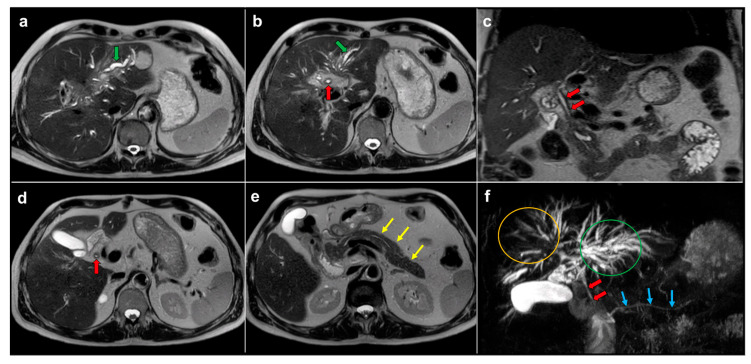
Primary sclerosing cholangitis in a male patient with ulcerative colitis and recurrent episodes of cholangitis. MRI including (a–e) axial and coronal T2WI and (f) coronal 3D MRCP with MIP reconstruction. Circumferential wall thickening and strictures of the CHD and CBD are observed (red arrows), together with short multifocal strictures of the intrahepatic bile ducts, resulting in a “pruned-tree” appearance on MRCP (orange circle). Note also the alternation of normal segments and saccular dilatations (green arrows), producing a “beaded” appearance on MRCP (green circle). The pancreas shows preserved morphology and signal intensity on T2WI (yellow arrows), and the MPD calibre remains normal on MRCP (blue arrows), with no imaging features suggestive of AIP.
